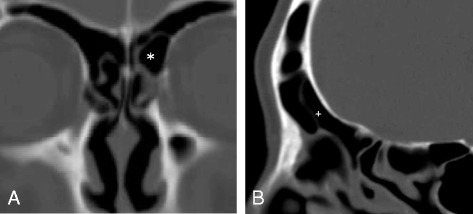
Computed tomography (CT) images of a healthy frontal sinus. a Coronal CT showing a left frontal cell type 3 (FC3) (*). b Sagittal CT showing a frontal bullar cell (FBC) (+)
